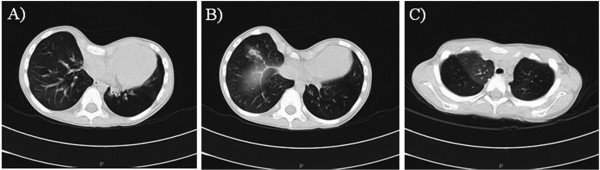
Chest CT scan before thoracic surgery. A) B) severe pectus excavatum with a Haller index of 4.07, bronchiectases and ground glass opacities C) area of consolidation and air trapping.
